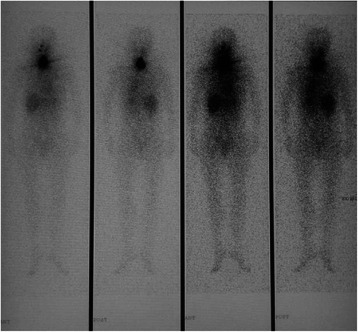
Emission Computed Tomography (ECT) 5 days after I^131^ treatment. As shown, I^131^ were uptaken mainly in the thyroid bed, but also in the liver, stomach, oral and nasal cavity.
